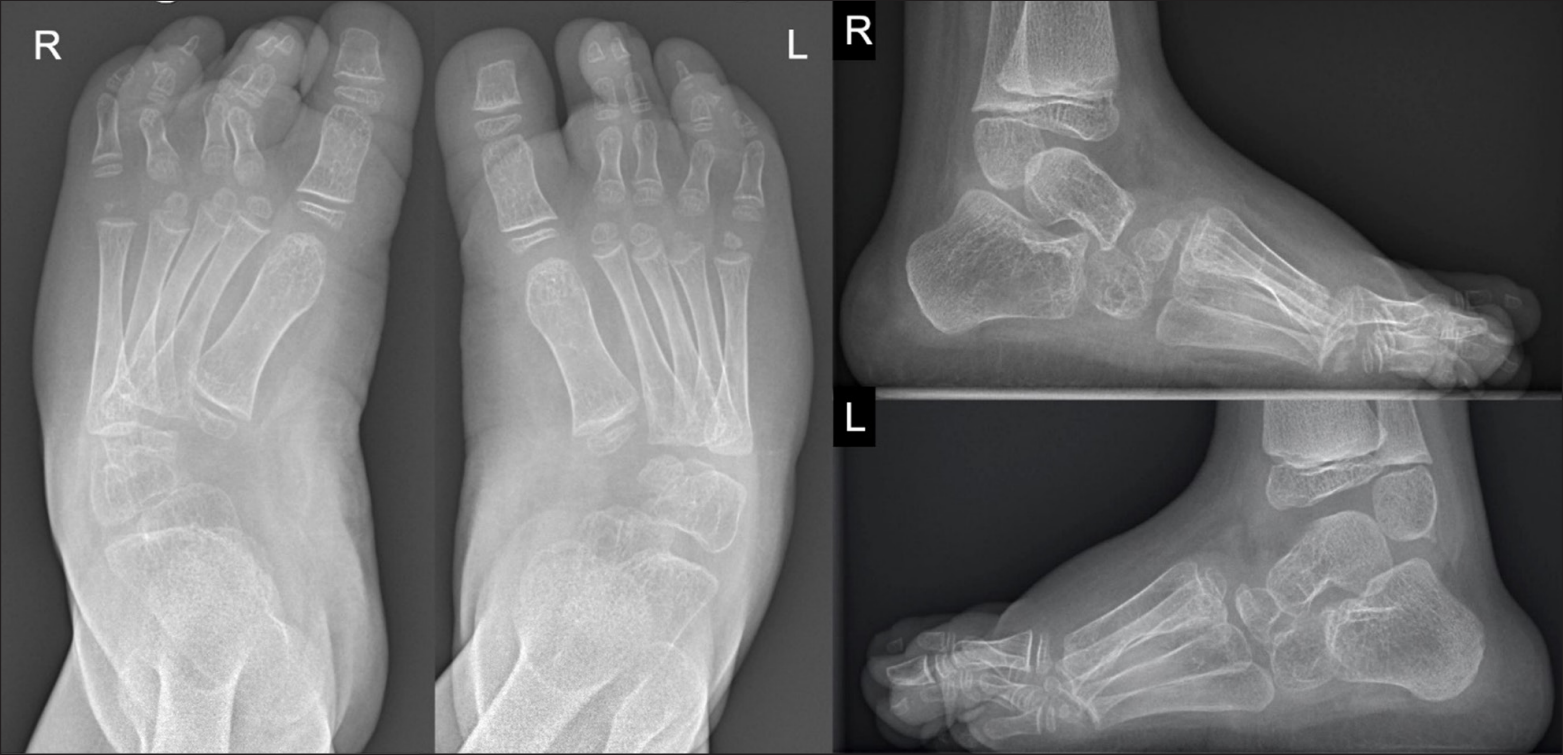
The radiographs of the ankle and feet reveal an extensive osteolysis of the tarsal bone, especially at the medial side of the midfoot and proximal metatarsal bone, causing a slight inward deformity of the forefeet.
